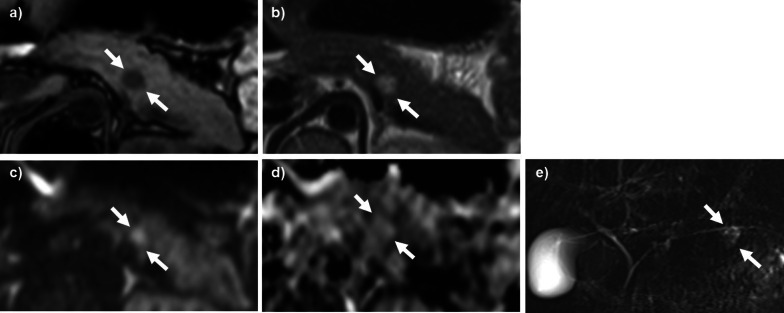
MRI of the abdomen. a T1WI, b T2WI, c DWI, d ADC-map, and e MRCP. An abdominal MRI revealed a homogeneous mass with low intensity on the T1-weighted image (T1WI), and high intensity on the T2-weighted image (T2WI) and diffusion-weighted image (DWI) and a slightly high intensity on the apparent diffusion coefficient-map (ADC-map) in the pancreatic tail (white arrows in a–d). A MRCP showed a high-intensity irregular lesion with slight indistinct margins without stenosis and dilatation of the main pancreatic duct in the tail of the pancreas (white arrow in e)
